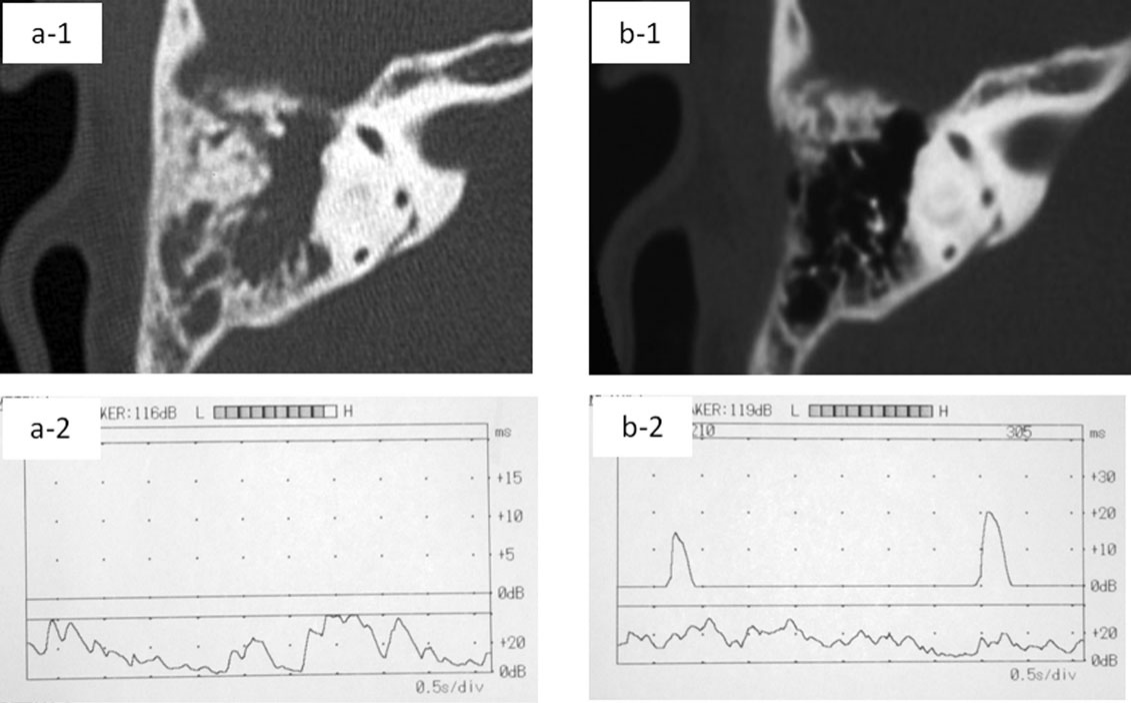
Typical case of improved Eustachian tube function after MAC regeneration. Nine-year-old female with cholesteatoma with adhesive otitis media. (a-1, b-1) High-resolution CT scan of the temporal bone. (a-2, b-2) Sonotubometry test results for Eustachian tube ventilatory function. (a) Pre-operation. Development of mastoid air cells and Eustachian tube function is poor and there is no aeration in the mastoid cavity. (b) Six months after the second-stage operation. Regenerated mastoid air cells and good aeration in the mastoid cavity are observed along with concurrent improvement of Eustachian tube function.
